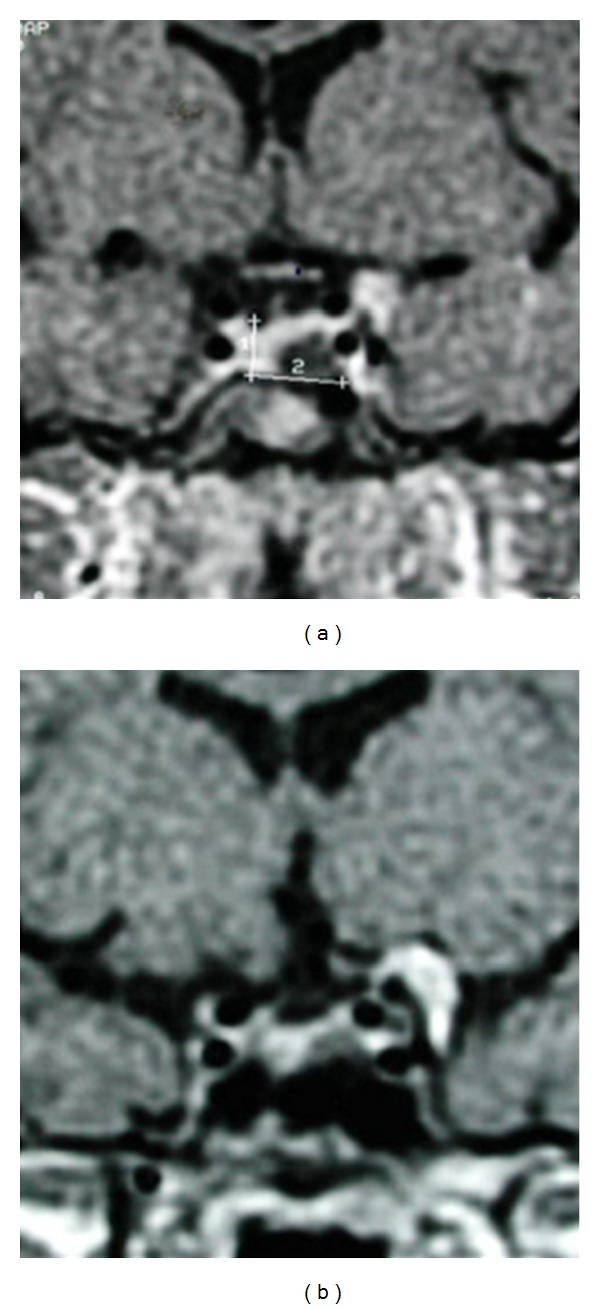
Ideal tumor for surgery. (a) Contrast enhanced MRI in coronal view showing a small tumor (line number 2) located on the left part of the sella turcica. Pituitary gland (line number 1) appears slightly displaced. (b) Postoperative result. In this case, a clinical and biochemical remission could be obtained.
